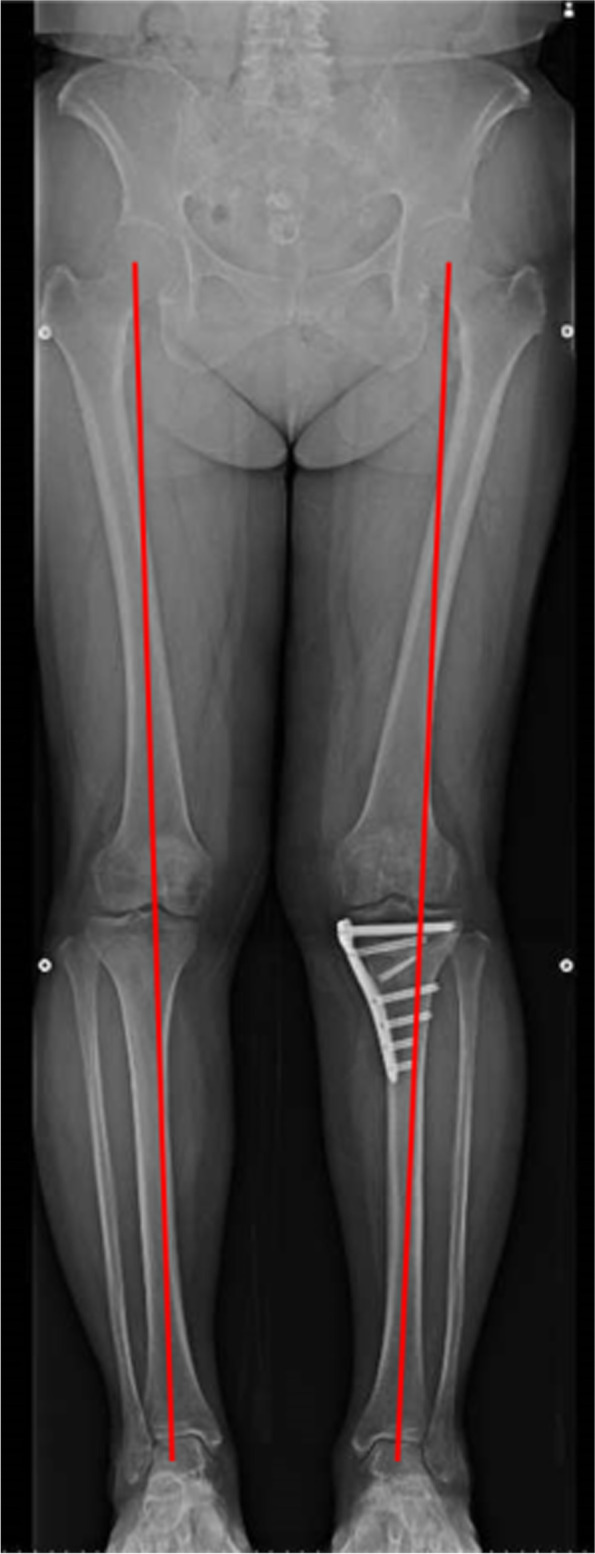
Radiographs after open-wedge high tibial osteotomy for neutral alignment. The red lines represent the mechanical axis of the lower limb on standing whole-leg radiograph. The correction angle of the left knee was 6 degrees
